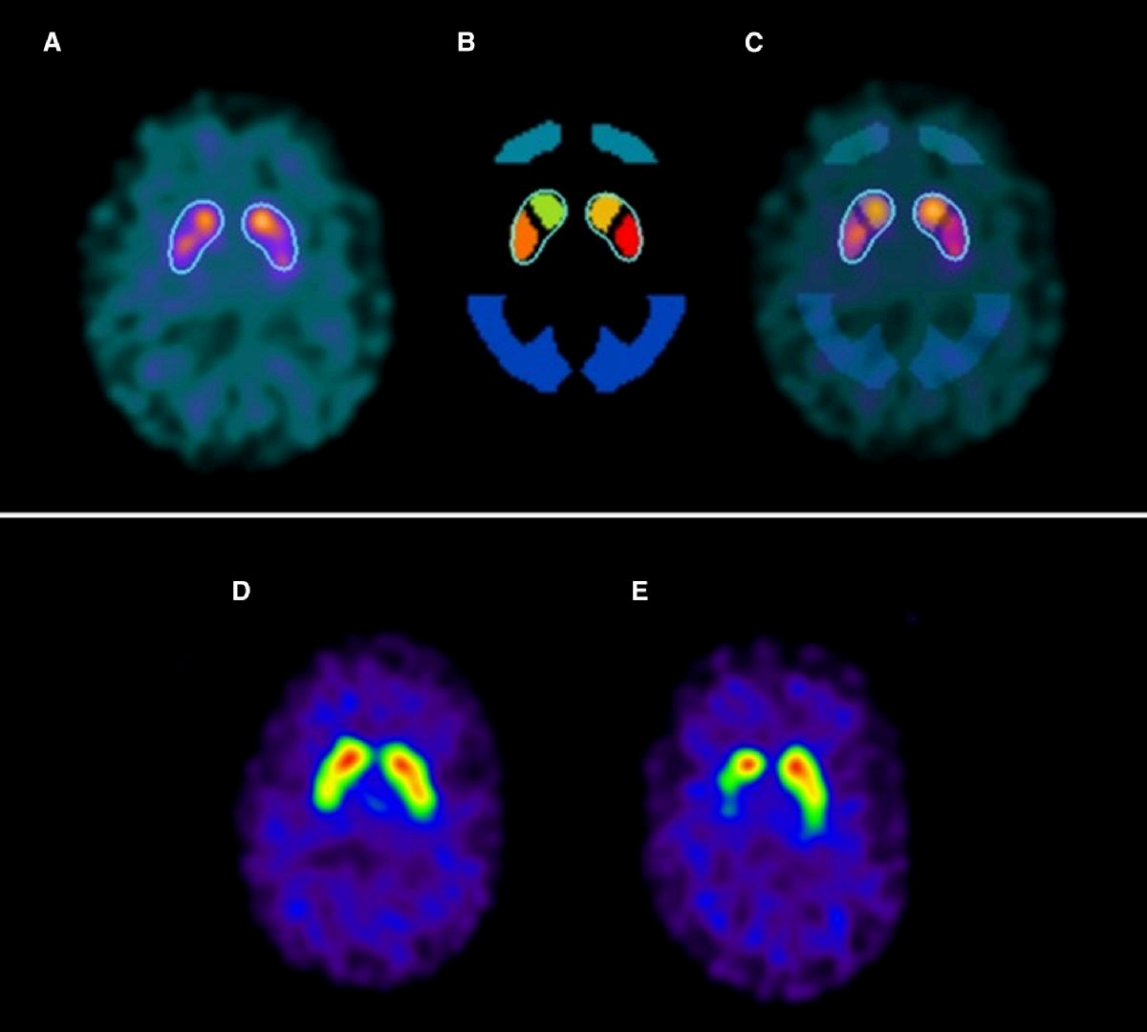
DaT SPECT/CT imaging in RBD patients. (A) Example striatal ROI registered to DaT SPECT/CT. (B) ROI used in the calculation of striatal SURs. Regions used were putamen [posterior ROIs within the striatal outline, shown in red (left putamen) and orange (right putamen)] and occipital reference (dark blue). (C) ROI superimposed on an example DaT SPECT/CT image. (D and E) Example DaT SPECT/CT images from two RBD patients with normal (D) and abnormal (E) imaging. The abnormal image (E) demonstrates asymmetric signal loss in the putamen, typical of Parkinson’s disease.
